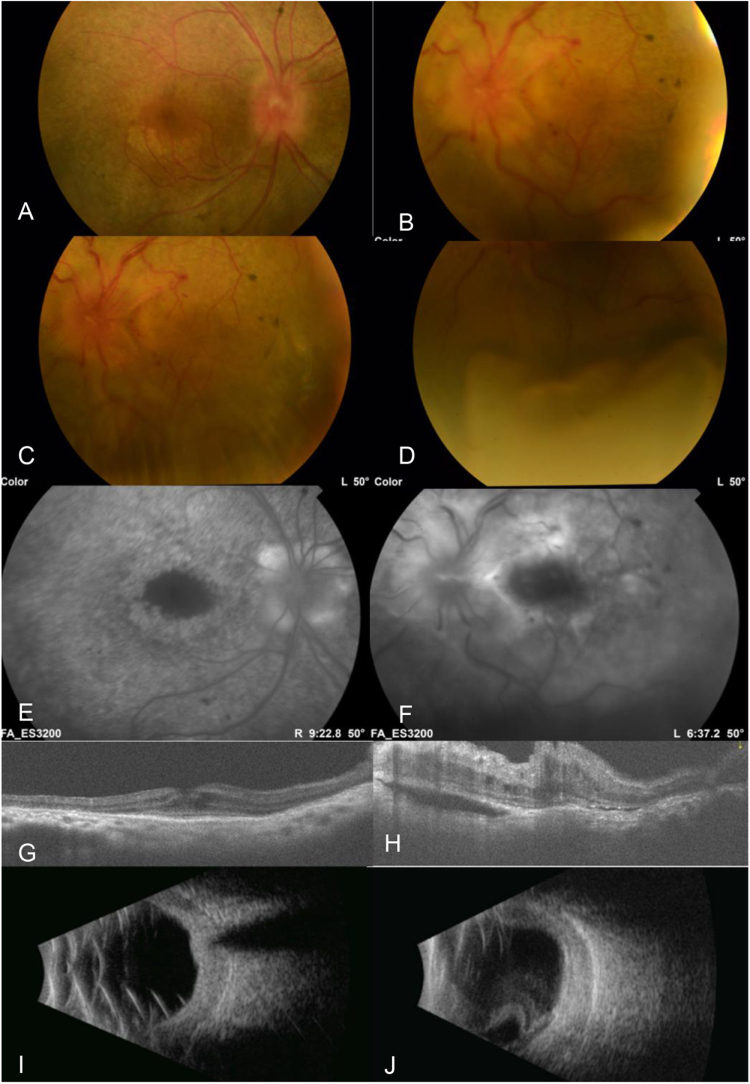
(A–C) Color fundus images showing bilateral optic disc swelling and macular thickening with peripheral pigmentary changes. The left eye exhibited more severe changes, including severe disc swelling. (D) Inferior exudative retinal detachment. (E–F) Fundus fluorescein angiography for both eyes–right (E)and left (F)–showing no signs of retinal or optic disc inflammation. (G–H) Spectral domain optical coherence tomography (SD-OCT) of both eyes showing severe subretinal and intraretinal fluids, as well as retinal wrinkles in the left eye (H). (I–J) B-scan ultrasonography showing bilateral retinochoroidal thickening and optic nerve head elevation in both eyes, with inferior retinal detachment extending from posterior to the equator and an intact superior retina in the left eye (J).
